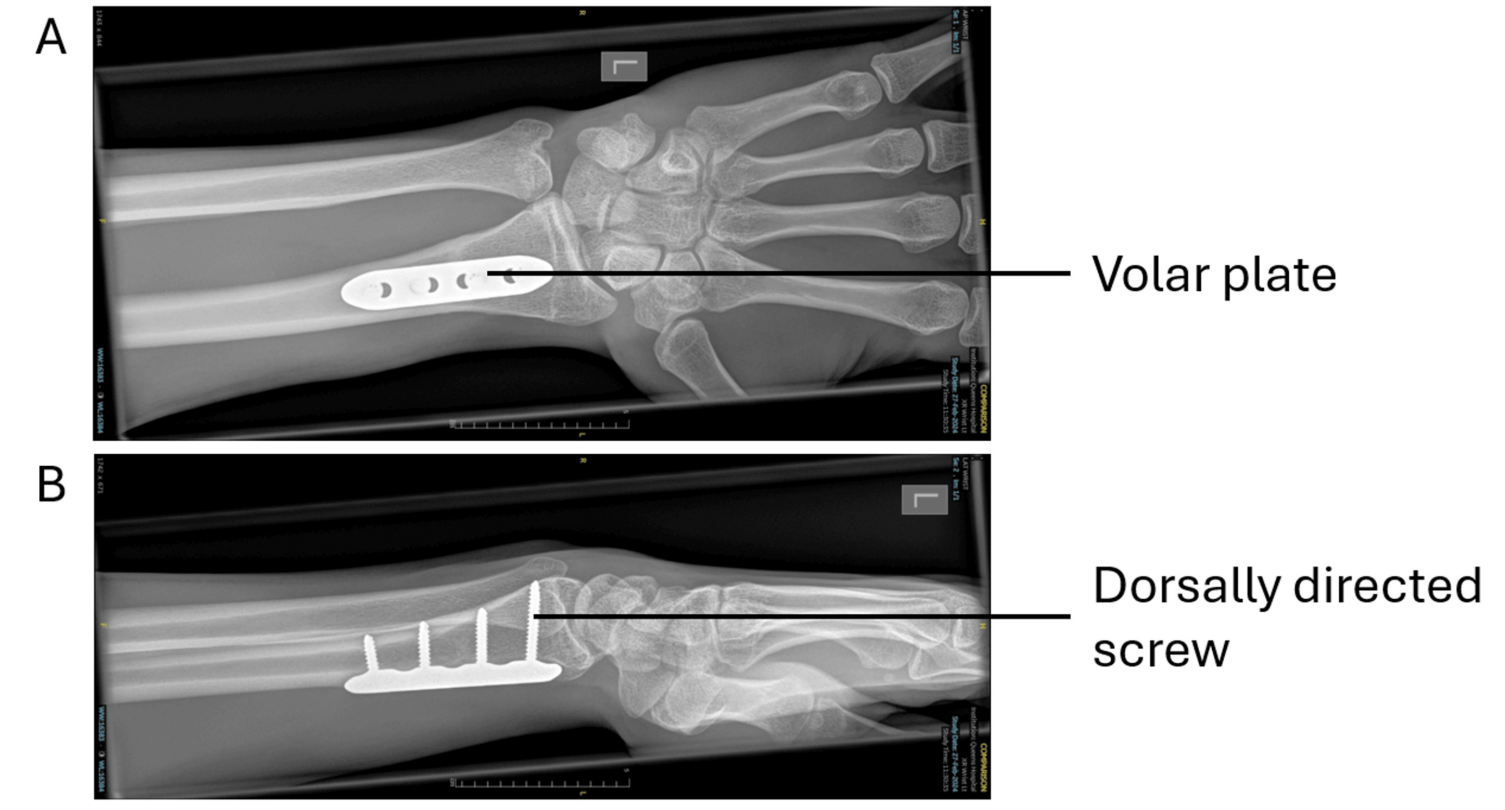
A) AP X-ray of the left wrist with a volar plate. B) Lateral X-ray of the left wrist with a volar plate.
